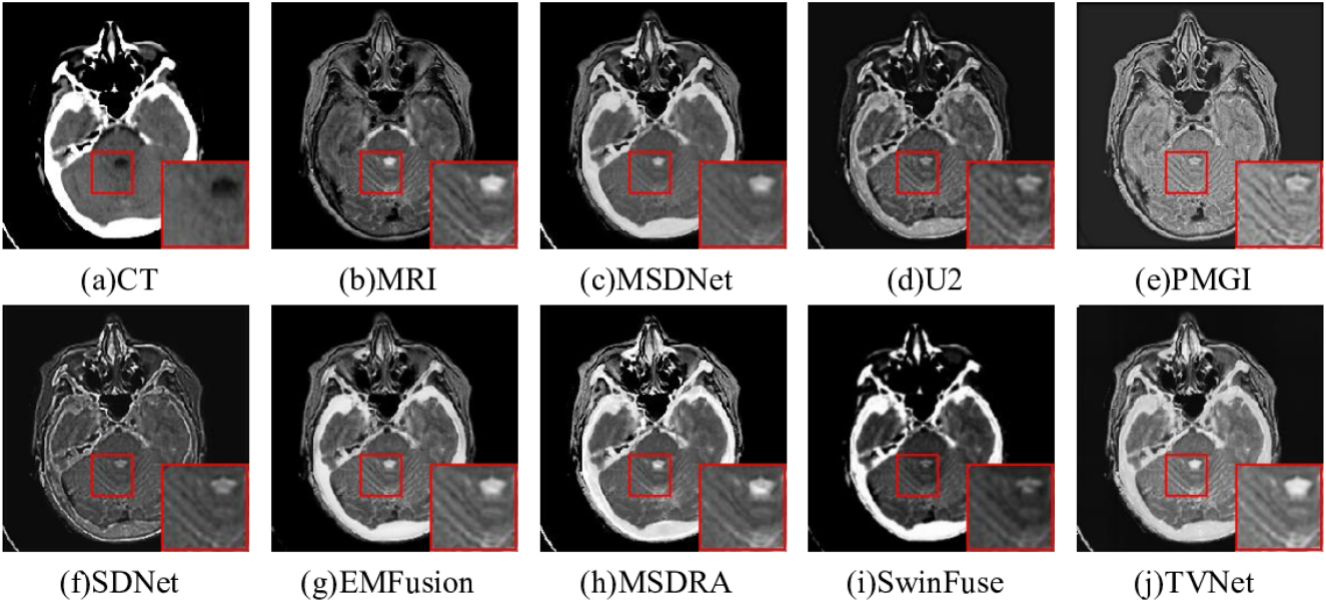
Comparison on computed tomography-magnetic resonance imaging (CT-MRI) fusion.
